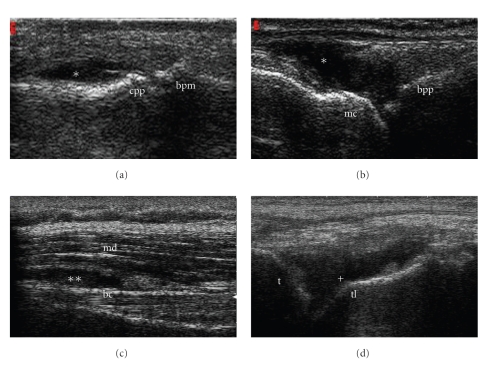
Longitudinal grey-scale ultrasound images of the third proximal interphalangeal joint from dorsal view with synovitis* (a), the third metacarpophalangeal joint from dorsal view with synovitis* (b), the shoulder joint from anterior view with subdeltoid bursitis, and the tibiotalar joint with effusion^+^ (d). Abbreviations; cpp: Caput of phalanx proximalis; bpm: Basis of phalanx medialis; bpp: Basis of phalanx proximalis; mc: Caput of metacarpus; md: Deltoid muscle; bc: Tendon of long biceps muscle; tl: Talus; t: Tibia.
